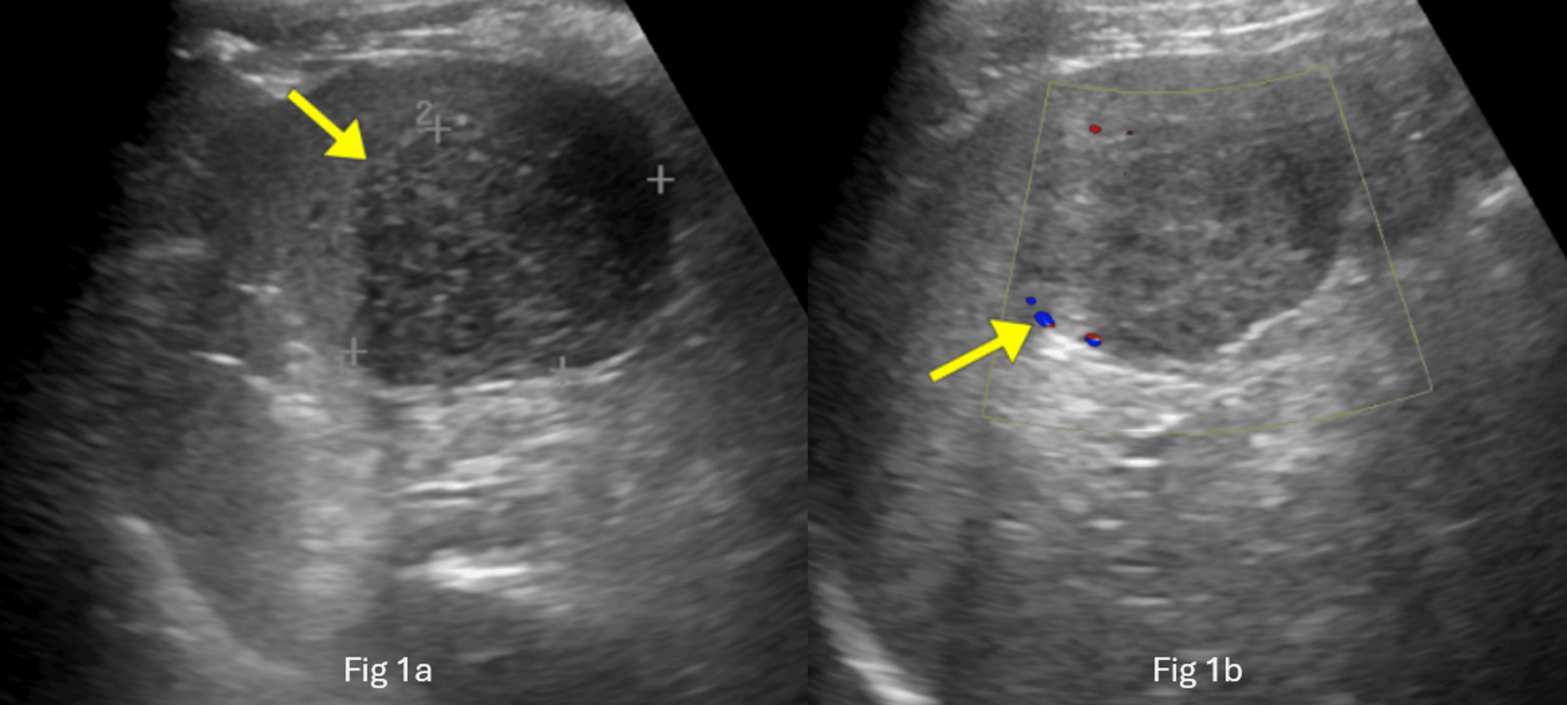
Ultrasound of the abdomen A large, well-circumscribed, heterogeneous lesion measuring 5.0 x 3.4 cm indicated by the yellow arrow in the lower pole of the spleen (1a), with only minimal peripheral flow on color Doppler (1b).
